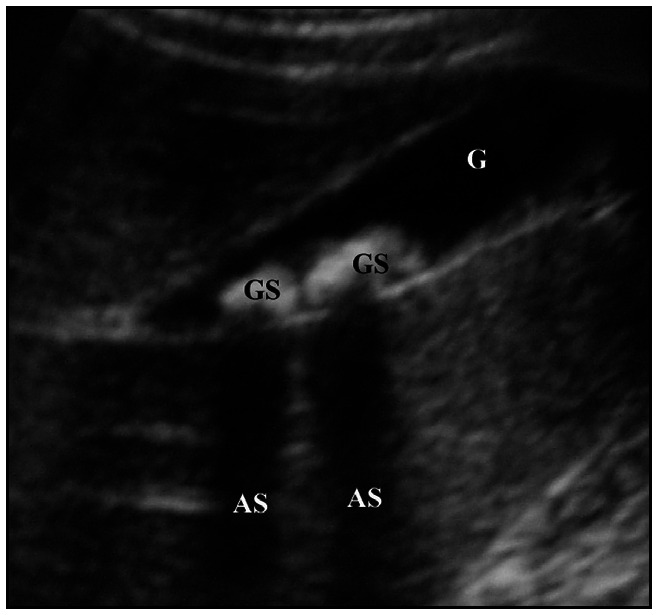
Ultrasound appearance of biliary lithiasis. G = Gallbladder, GS = Gallstones, AS = Acoustic shadow.
